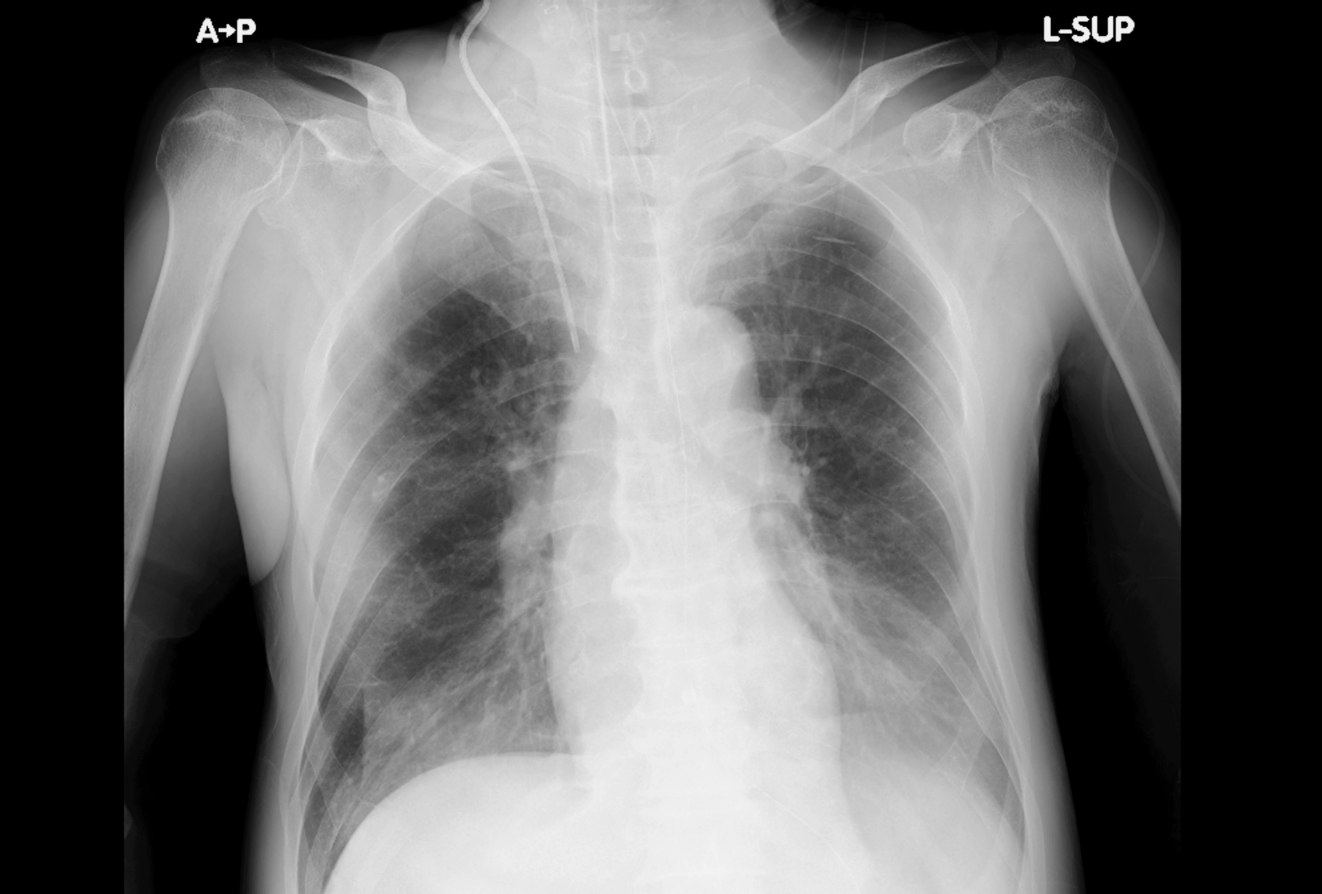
Chest X-ray on day 25 The chest X-ray on day 25 also showed improvement.
